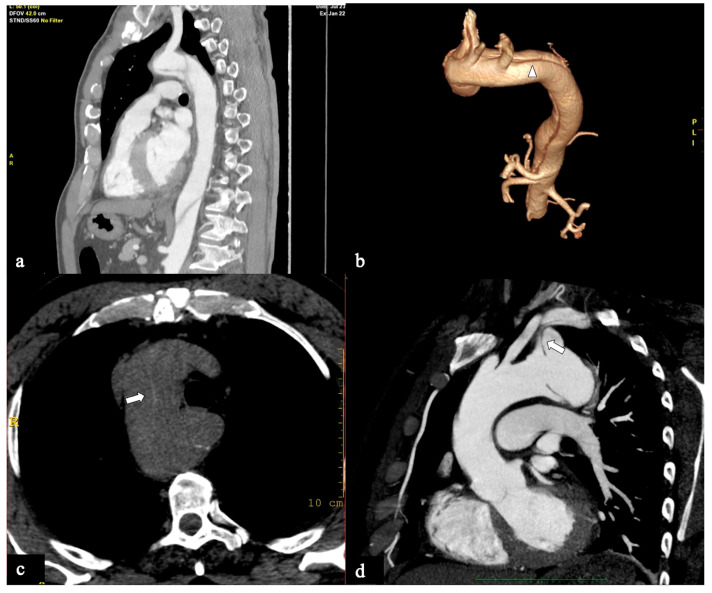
CTA images of non-A–non-B dissection with descending entry and retrograde arch extension or Configuration 1 (DTA entry). (a) Sagittal MIP and (b) volume-rendering CTA reconstructions in a 74-year-old male showing Configuration 1 dissection with retrograde flap (arrowhead) propagation along the arch curvature. (c) In a 65-year-old male patient unenhanced axial CT image shows a hyperdense propagated flap in a right aortic arch (arrow). (d) In a 68-year-old female patient curved MIP CTA reconstruction shows left subclavian artery involvement (arrow) in a Configuration 1 dissection.
